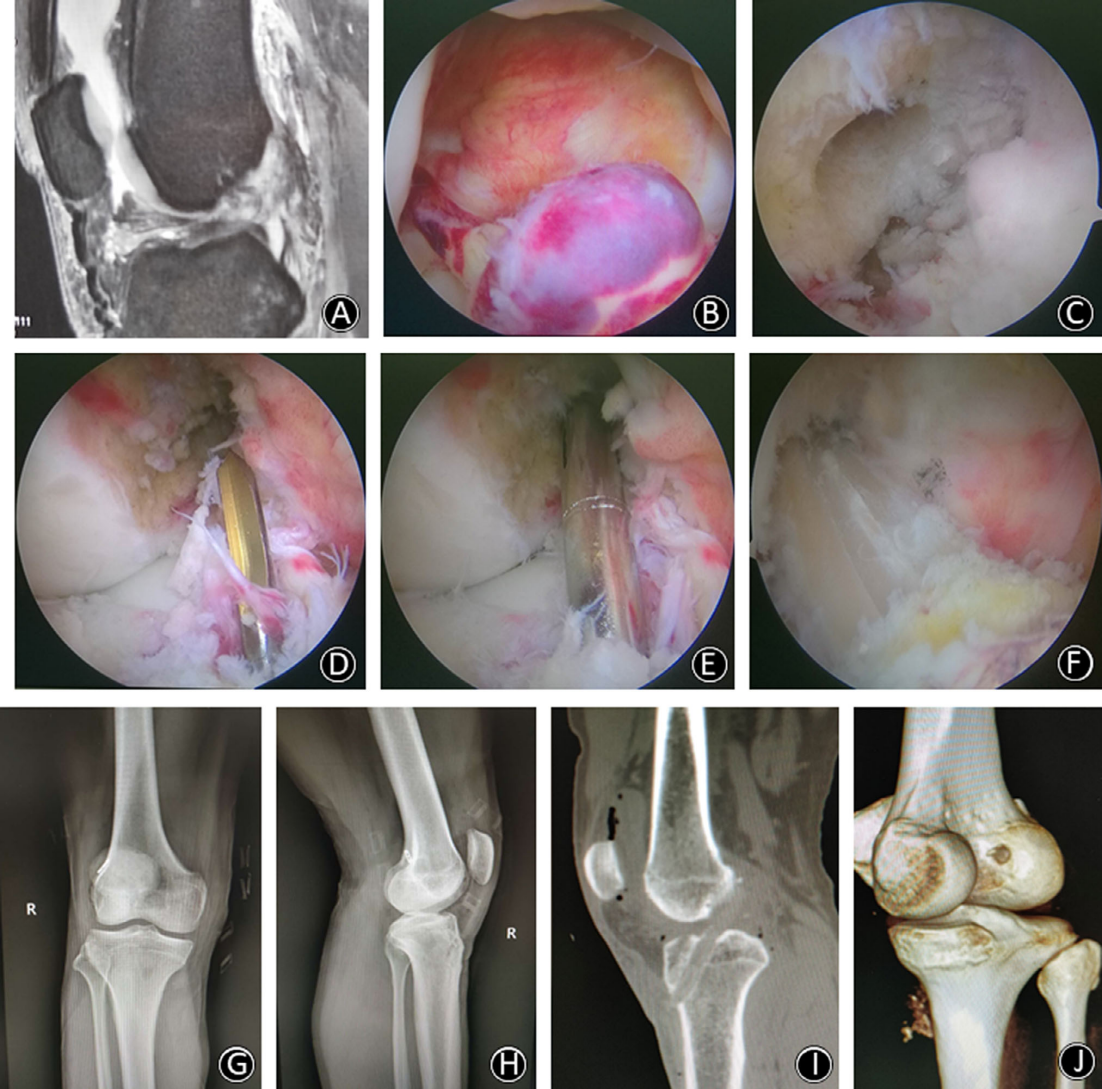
(A) MRI showed that ACL was broken, and the fiber of ACL was not continuous in the joint. (B) ACL rupture could be observed under arthroscopy, and ACL remnant showed cyclops lesion in the knee. (C) The position of the I.D.E.A.L. tunnel after the creation of the femoral tunnel. (D) The position of Kirschner wire in the tibial tunnel was located at the center of ACL remnant. (E) The shaver could reach the femoral tunnel through the tibial tunnel. It can be seen that the correspondence between the positions of the two tunnels in the joint was appropriate. (F) The graft of reconstructed ACL was well positioned in the joint. (G,H) Postoperative anteroposterior and lateral plain radiographs showed that the TightRope button was flipped on the lateral cortex of the distal femur by the distal traction applied to the graft. (I) The tibial tunnel (sagittal CT image) was located at the anatomic position. (J) 3D CT scan reconstruction with a femoral tunnel position.
